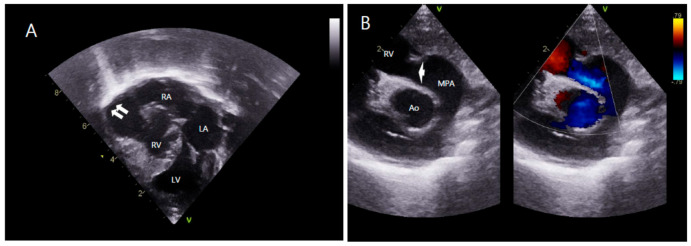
Transthoracic echocardiogram. (A) Apical four-chamber view shows aneurysmal dilatation of RV (white arrow) with apical muscular hypertrophy. (B) Parasternal long-axis view shows absence of pulmonary valve leaflets (white arrow) with free pulmonary regurgitation. LA, left atrium; LV, left ventricle; RA, right atrium; RV, right ventricle; Ao, aorta; MPA, main pulmonary artery.
